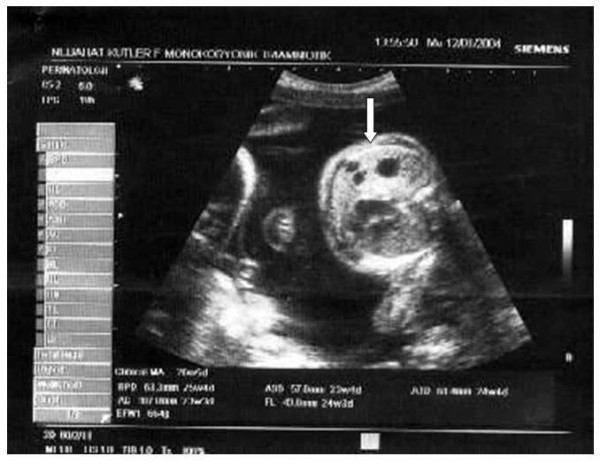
Transabdominal ultrasonography is presenting the co-triplet fetus with multicystic lung lesions, suggesting congenital cystic adenomatoid malformation (arrow) at 24 weeks.
